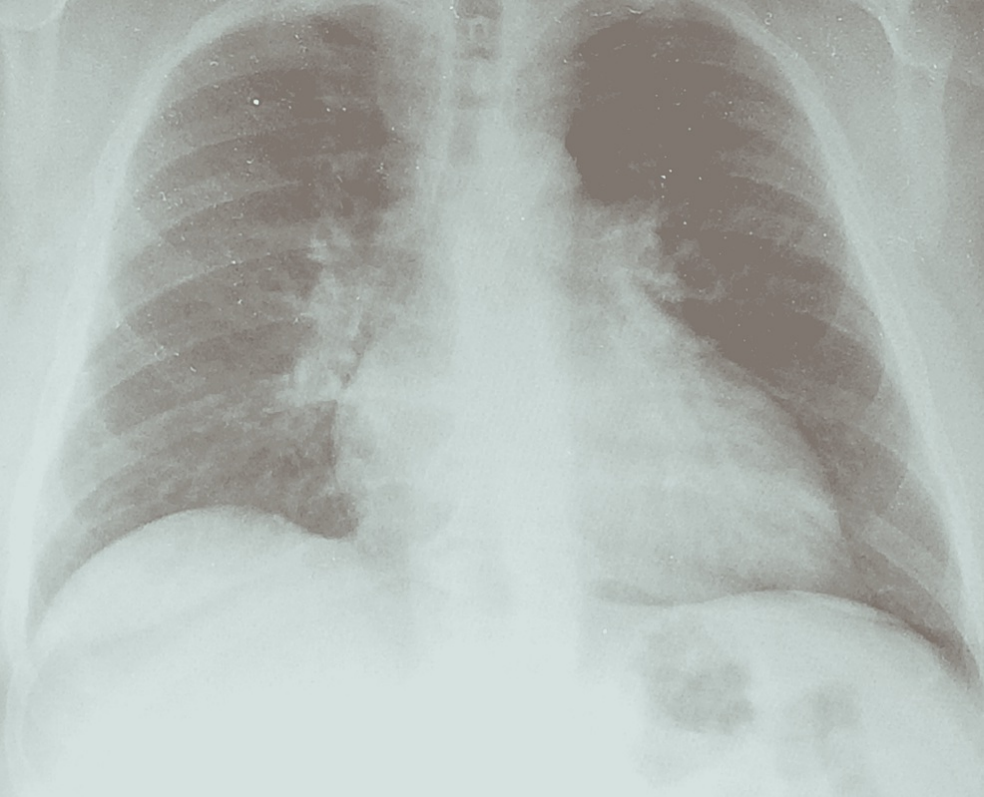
Chest X-ray showing mild pulmonary edema
